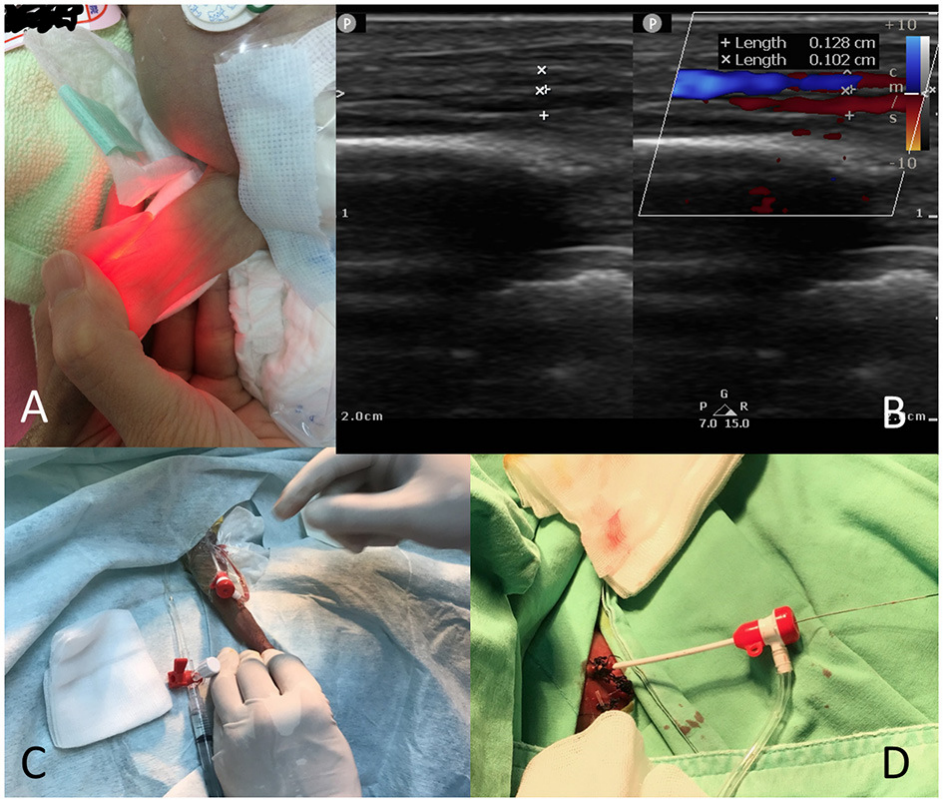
Preparation of venous cannulation. (A) Use of a transilluminator to identify the femoral vein location in a 533-g baby; (B) Use of ultrasound to identify the femoral vein in a 509-g baby; (C) A 4F sheath (femoral vein) in a 533-g baby; (D) A 4F sheath (umbilical vein) in a 478-g baby.
